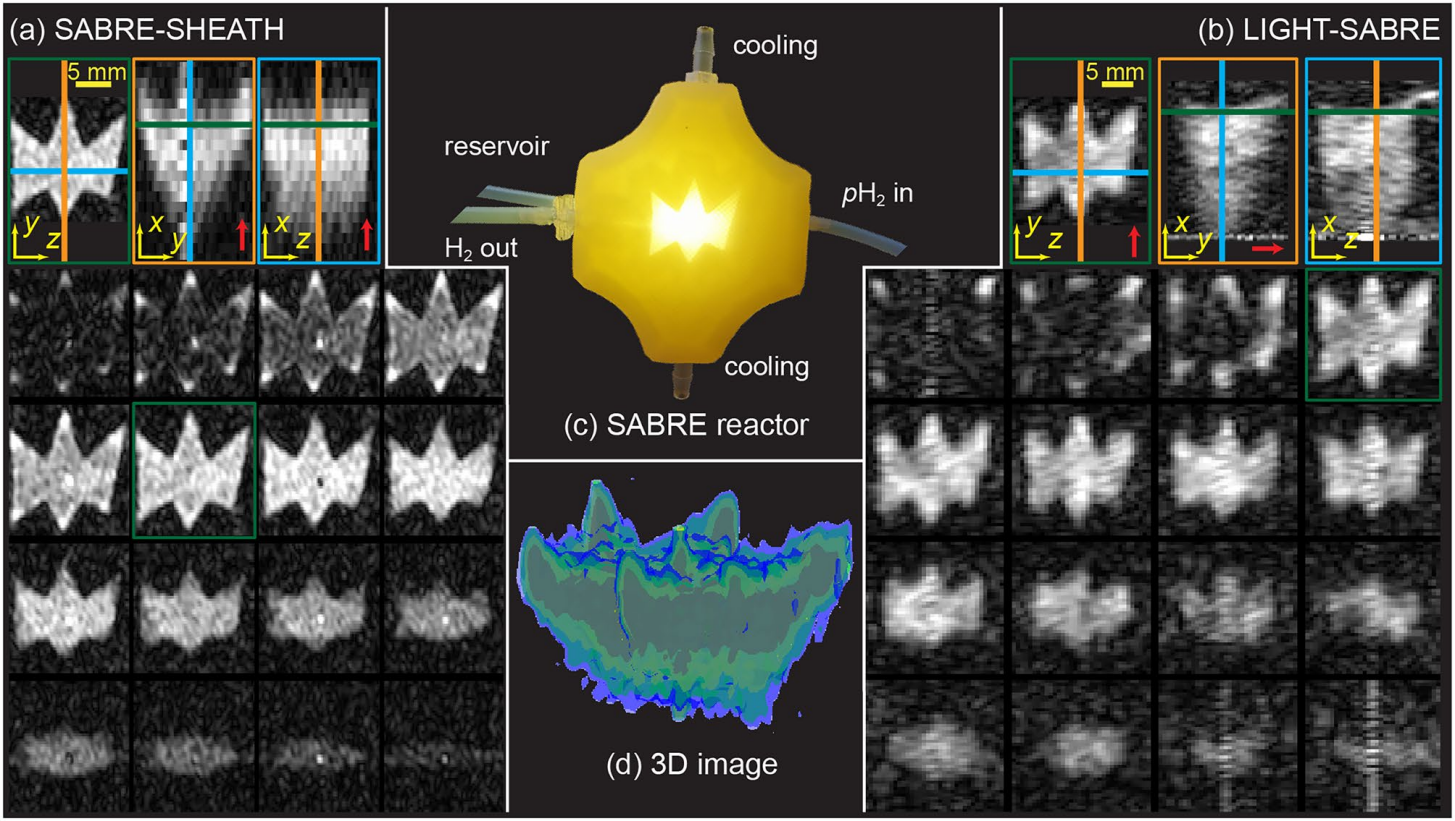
^13^C ULF MRI of hyperpolarized [1-^13^C]pyruvate in a SABRE reaction chamber. 3D spin-echo image of [1-^13^C]pyruvate polarized with SABRE-SHEATH (a) or LIGHT-SABRE (b) HP sequence. Red arrows indicate the frequency encoding direction. Photograph of 3D printed SABRE reaction chamber, illuminated from underneath (c). 3D multi-isosurfaces image acquired with SABRE-SHEATH (d).
